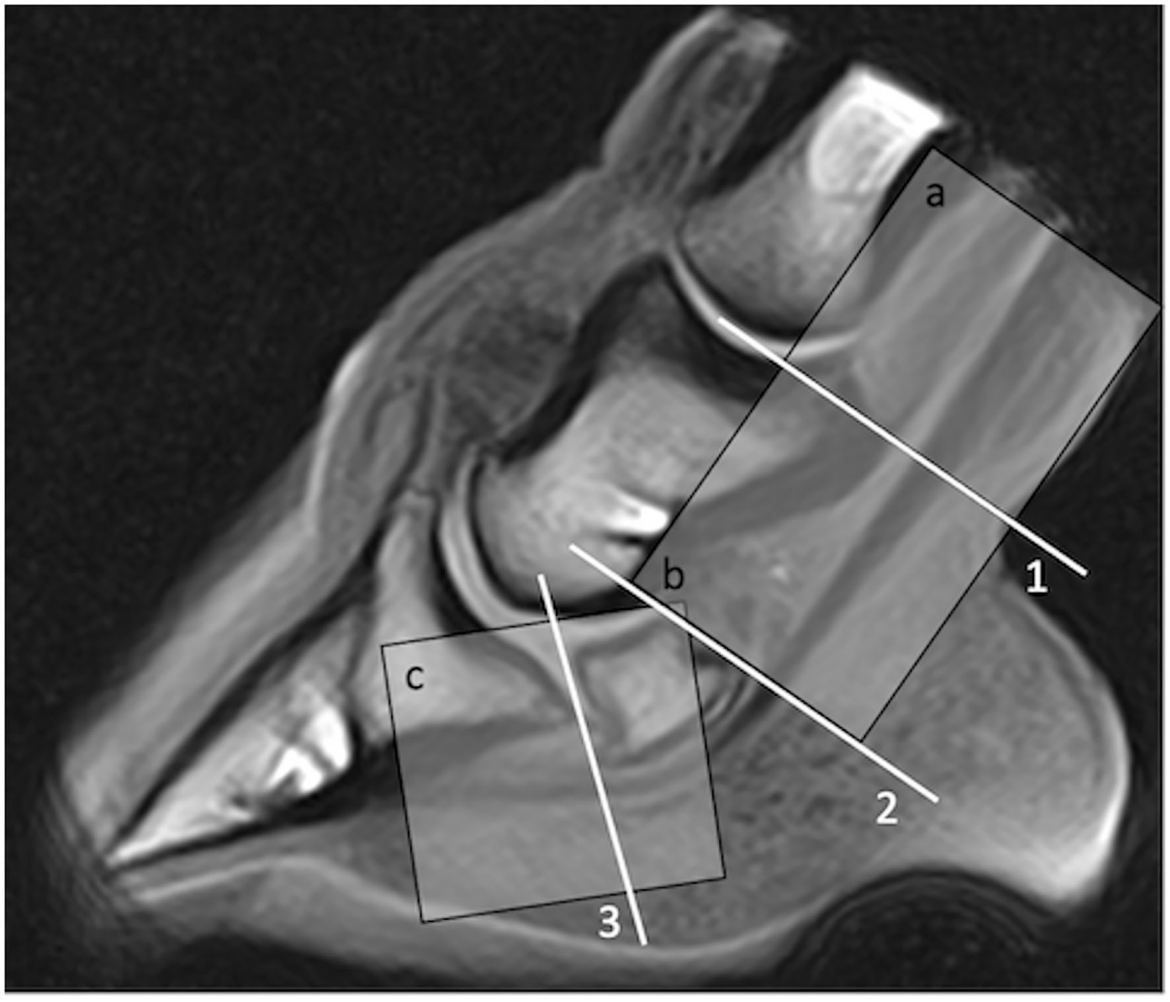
Proximo-distal levels used to describe deep digital flexor tendon lesion location at magnetic resonance (MR) imaging (1, suprasesamoidean; 2, sesamoidean; 3, infrasesamoidean) and at ultrasonography (a, pastern; b, suprasesamoidean; c; distal sesamoidean and infrasesamoidean explored together with transcuneal approach). Region a (pastern) was not imaged using MR in this study.
